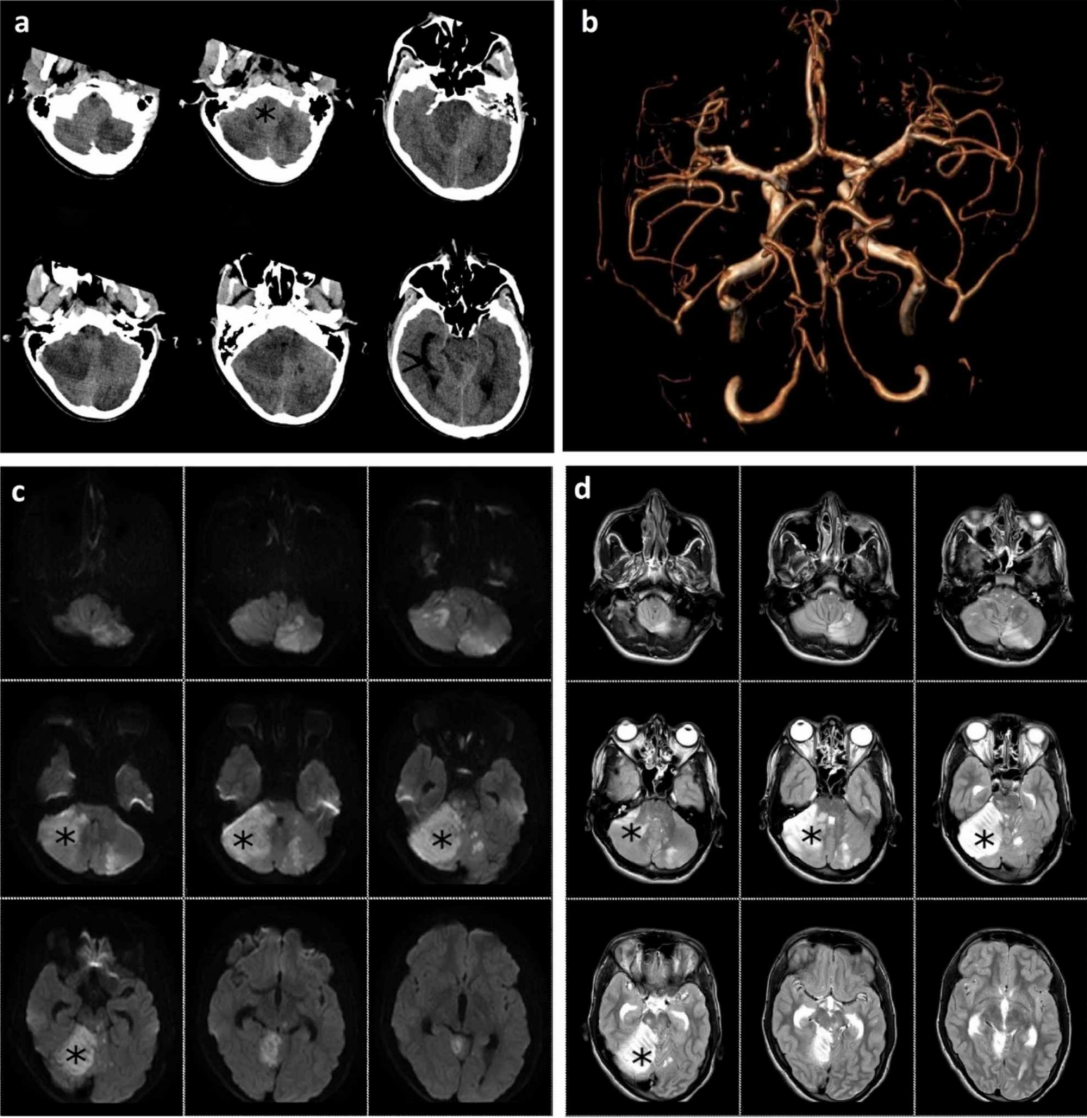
Computed tomography (CT) and magnetic resonance imaging (MRI) examination after endovascular treatment a) Six hours after treatment, a CT scan showed the previously diagnosed ischaemic outbreaks in addition to initial dilatation of the supratentorial ventricular system (black arrowhead) and a reduction of the Silvio aqueduct and the fourth ventricle (black star), due to cerebral cytotoxic oedema; b) 24 hours after the endovascular procedure, magnetic resonance angiography (MRA) showed typical posterior circulation; c) in diffusion-weighted imaging (DWI) sequence and d) in fluid-attenuated inversion recovery (FLAIR) sequence, the ischaemic lesion (black stars) is evident with the same findings as those seen in the last CT scan. Immediately after MRI, a preventive occipital craniectomy was performed.
